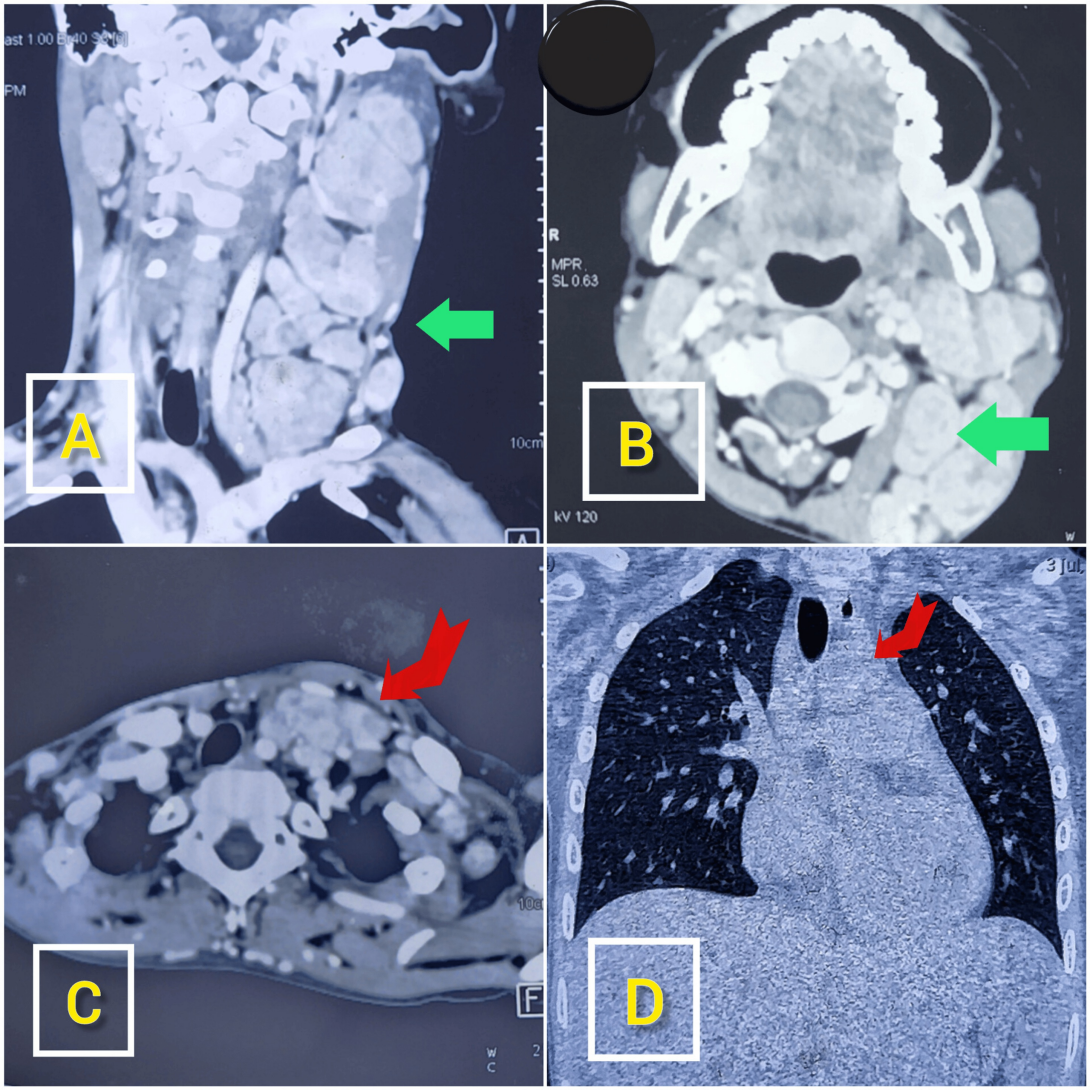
Contrast-enhanced computed tomography (CECT) Neck and Thorax showed multiple enlarged lymph nodes Multiple enlarged conglomerated lymph nodes (green arrows) in left level IB, II, III and IV in coronal section (A) and transverse section (B) of neck. Multiple enlarged lymph nodes in neck space extending up to the upper mediastinum (red angular arrows) in transverse section (C) and coronal section (D).
